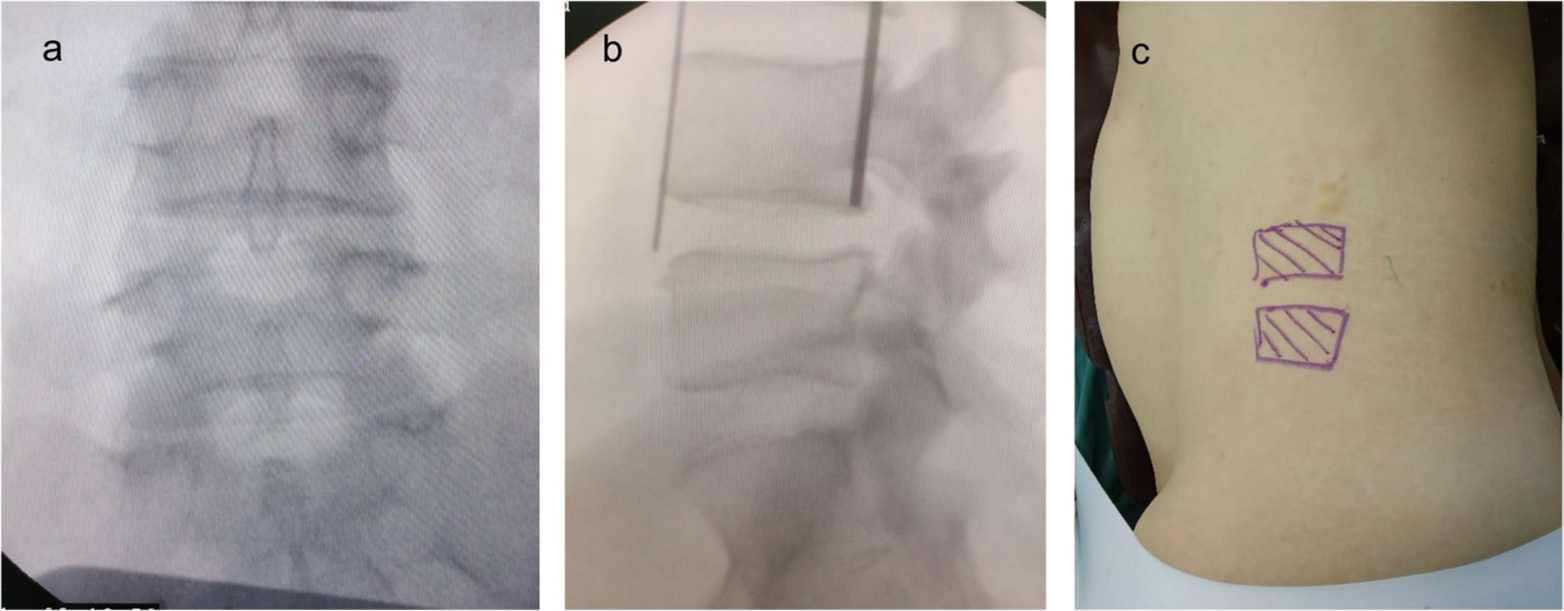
The operative segment is confirmed to be in absolute lateral position under fluoroscopy and the surface location is marked. a The spinous process is located in the center between two pedicles in anteroposterior X-ray. b The upper endplate of caudal vertebrae overlaps in a line and the affected disc space is marked with 2 K-wires in lateral X-ray. c The surface location of the affected disc space is marked on the patient’s lateral side
